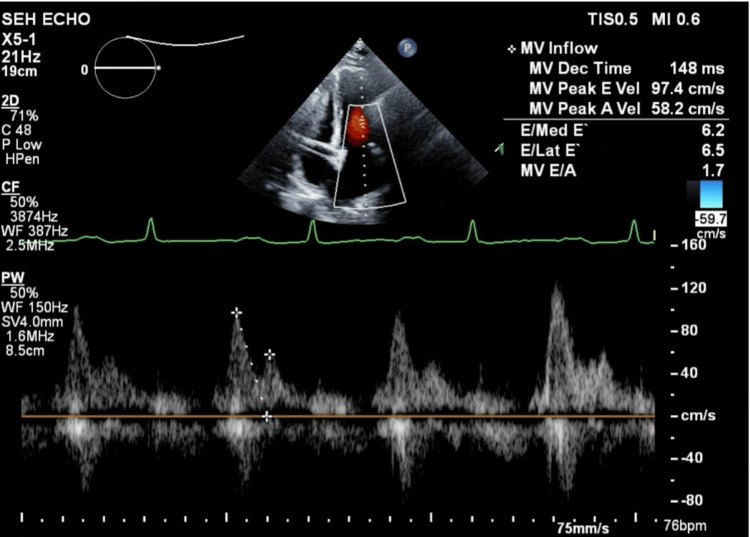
Transthoracic echocardiography showed preserved ejection fraction with mitral E/A ratio of 1.7 (greater than 1.5).
